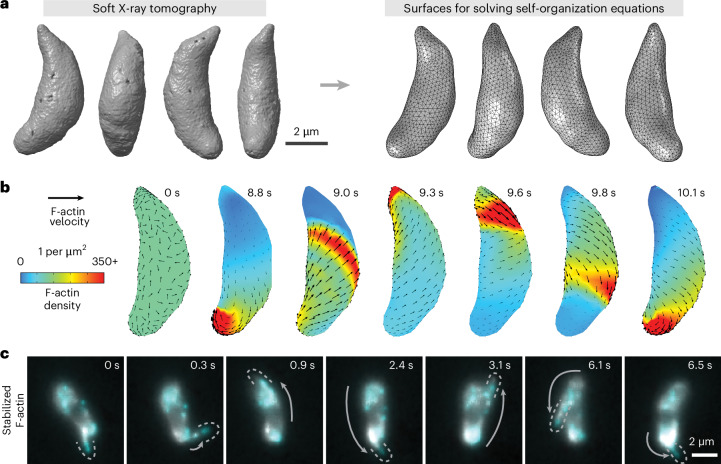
Stable actin filaments circle the *Toxoplasma* cell. a, Soft X-ray tomograms of cryo-fixed extracellular *Toxoplasma gondii* tachyzoites were used to generate triangle-meshed surfaces on which to solve our actin self-organization theoretical model. b, For stable filaments, solving the model constrained to *Toxoplasma*’s surface geometry predicts recirculating actin patches. c, In experiments, actin filaments briefly stabilized with jasplakinolide can circle around the cell. Cyan and grey both show actin, labelled at different dye densities. Images denoised with noise2void^60^. Dotted lines outline protruding actin filaments, and grey arrows highlight the movement of the protrusion since the previous frame. Representative of *n* = 10 cells whose protrusion velocities are characterized in Supplementary Fig. 4.
